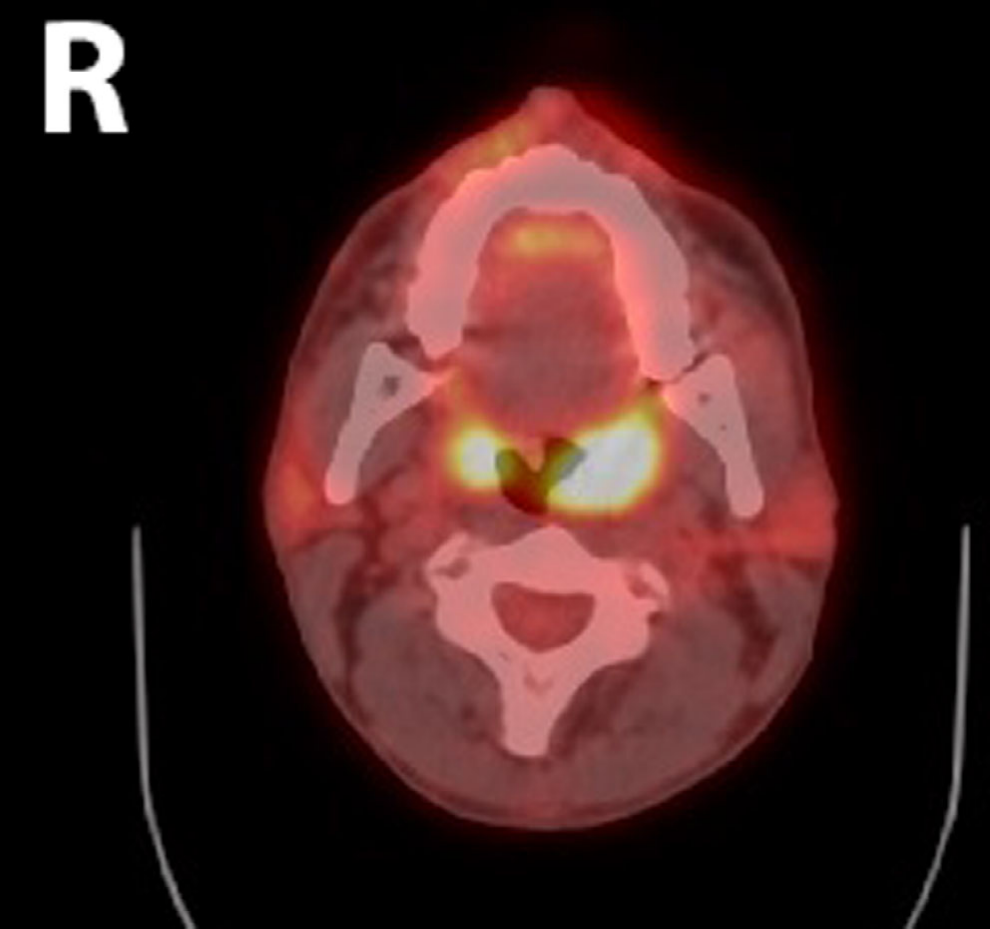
Whole body fluorodeoxyglucose (FDG)‐PET/computed tomographic (CT). Axial view showing increased radiotracer uptake in both the left and right palatine tonsils with SUV of 8.2 and 6.6, respectively
